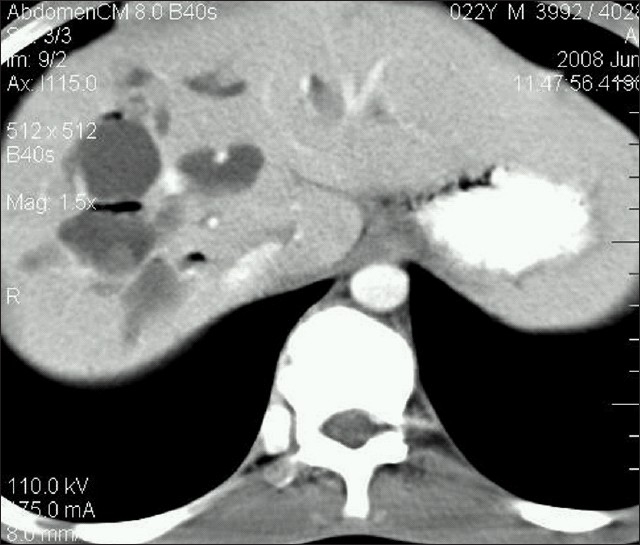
Contrast-enhanced computed tomography of abdomen showing dilated intrahepatic biliary ducts with air foci and presence of “central dot” sign representing intraluminal portal vein in dilated intrahepatic bile ducts in right lobe of liver
